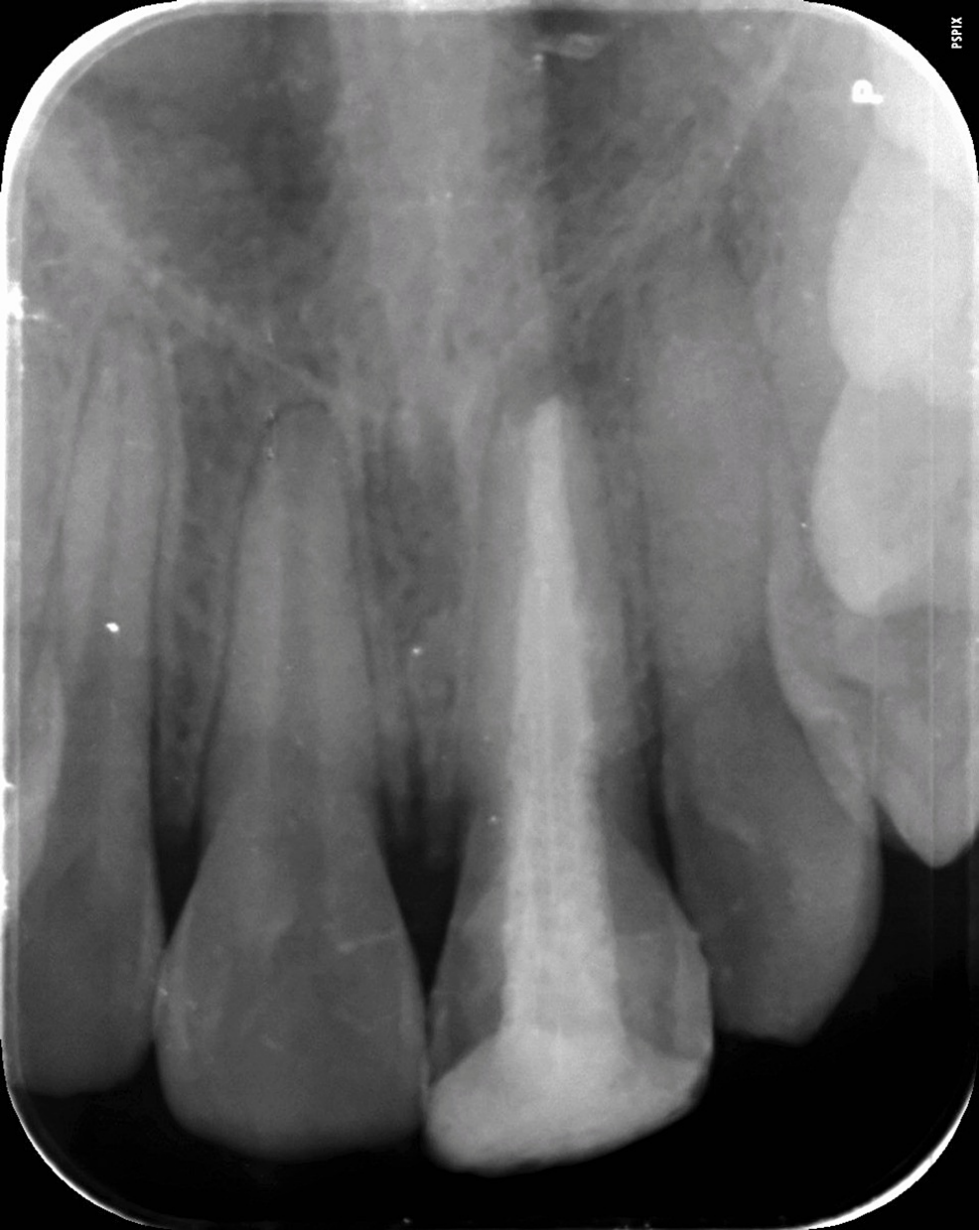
Radiographic picture of post cementation and core build-up with 21
